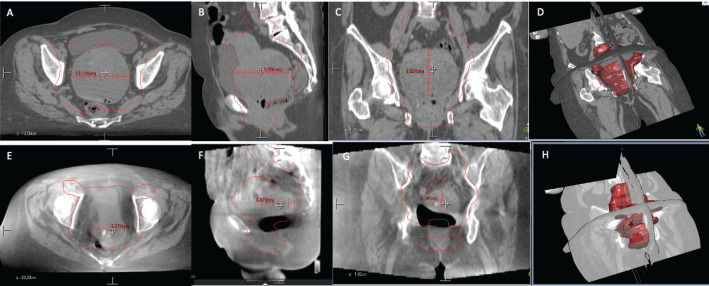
Comparison of CT images. (a–d): (Upper row, a–d) Before radiotherapy, uterus measures were 85.2 × 95.8 x 10.4 mm. (e–h): (Lower row, e–h) After radiotherapy, uterus measures were 43.4 x 60.7 x 62.7 mm.
